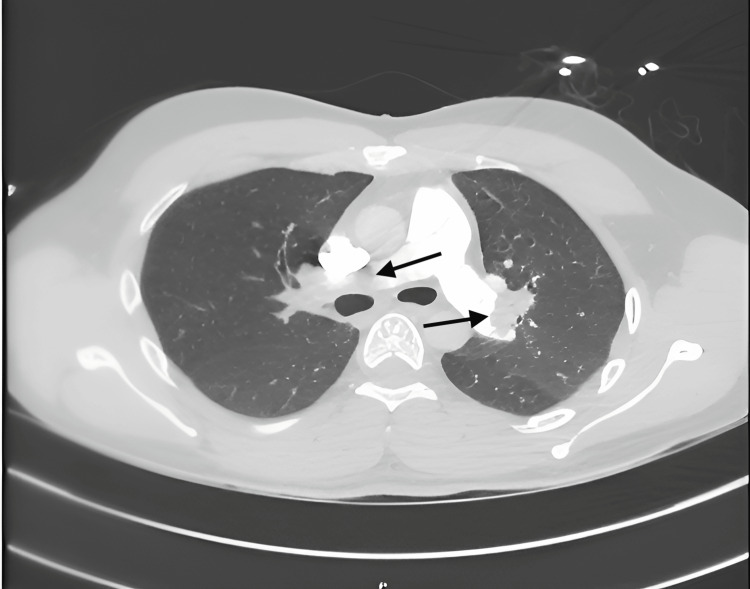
CTA demonstrating massive pulmonary embolism completely obstructing the left pulmonary artery and partially obliterating the right pulmonary artery CTA: computed tomography angiography
